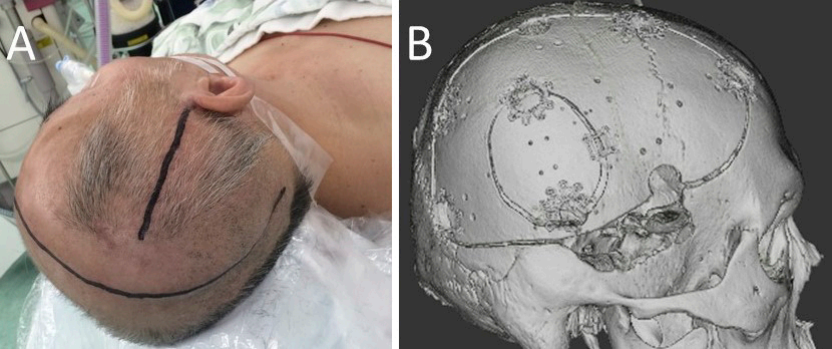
Photograph shows skin incision lines during small craniotomy and large decompressive craniectomy (A). Three-dimensional computed tomography image after cranioplasty (B).
